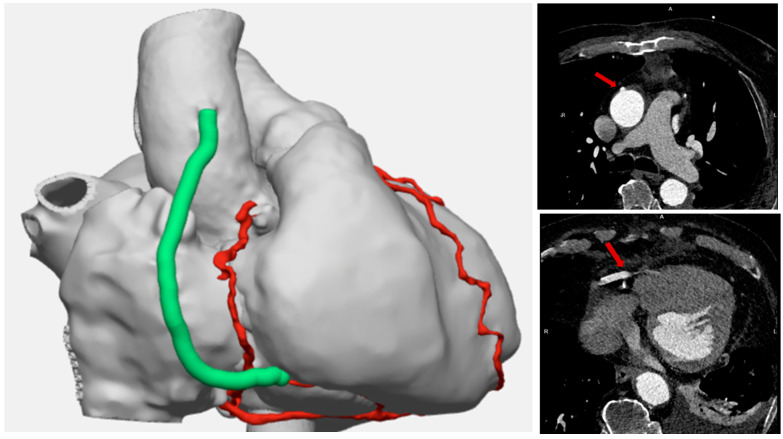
3D model rendering of the proximal and distal anastomosis (green conduit). CT scan showing the proximal and distal anastomosis (red arrows).
